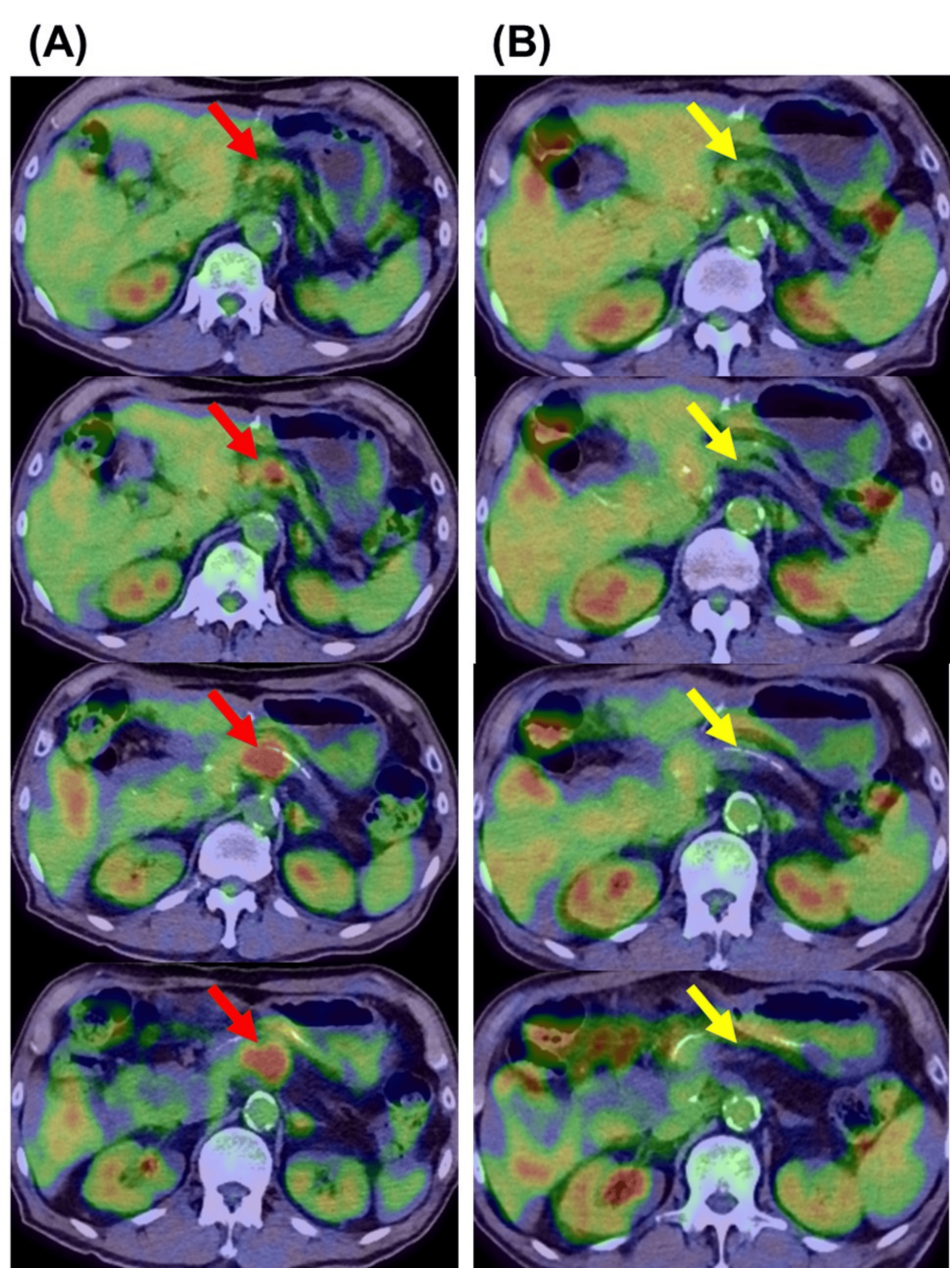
Positron emission tomography-computed tomography (PET-CT) examinations (A) before and (B) after the combined immune therapy Red arrows demonstrate the region of recurrent pancreatic cancer identified by PET-CT, and yellow arrows, performed after the immune cell therapy, demonstrate where the region of recurrent pancreatic cancer existed. The adjacent left and right images show the slices at the same layer.
